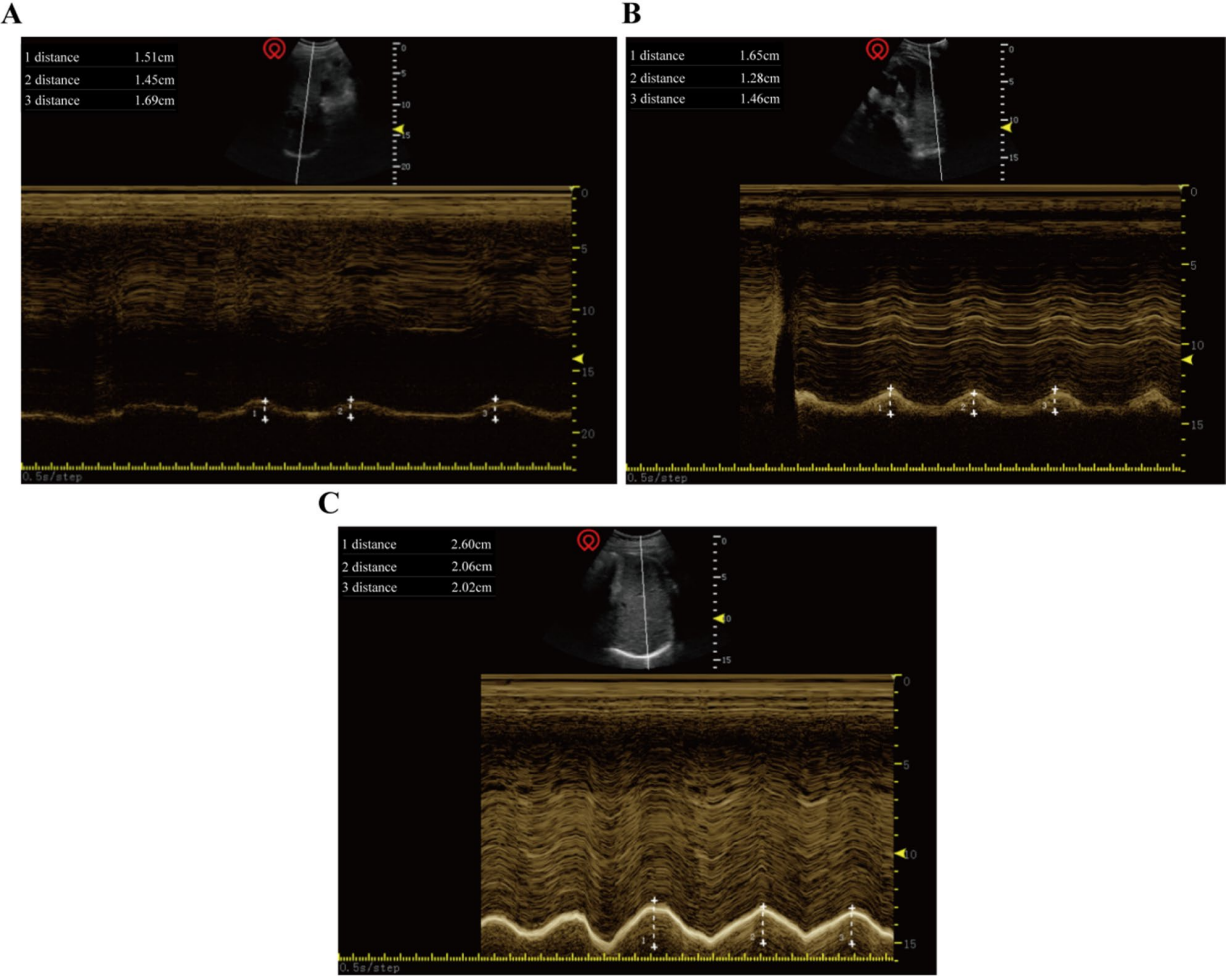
Ultrasound images of the left and right diaphragms in M-mode and measurement of diaphragmatic excursion. A Ultrasound image of the right-side diaphragm (liver acoustic window); B Ultrasound image of the right-side diaphragm (Splenic acoustic window); C Measurement of diaphragmatic excursion under M-mode ultrasound. Note: When three consecutive stable waveforms appear in the DE waveform shown by M ultrasound, the distance from the baseline to the crest of the waveform is measured and the average value (shown as 1, 2, and 3 in the figure) is recorded as the DE
